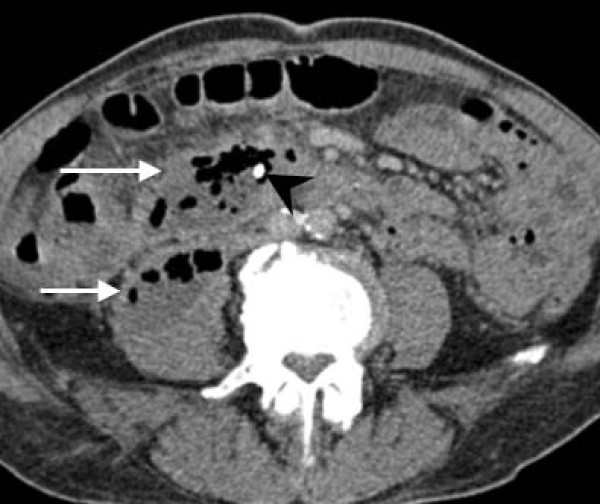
Axial computed tomography image. Large, gas-filled retroperitoneal abscess (white arrows) with the tip of the stent inside the collection (arrowhead).
